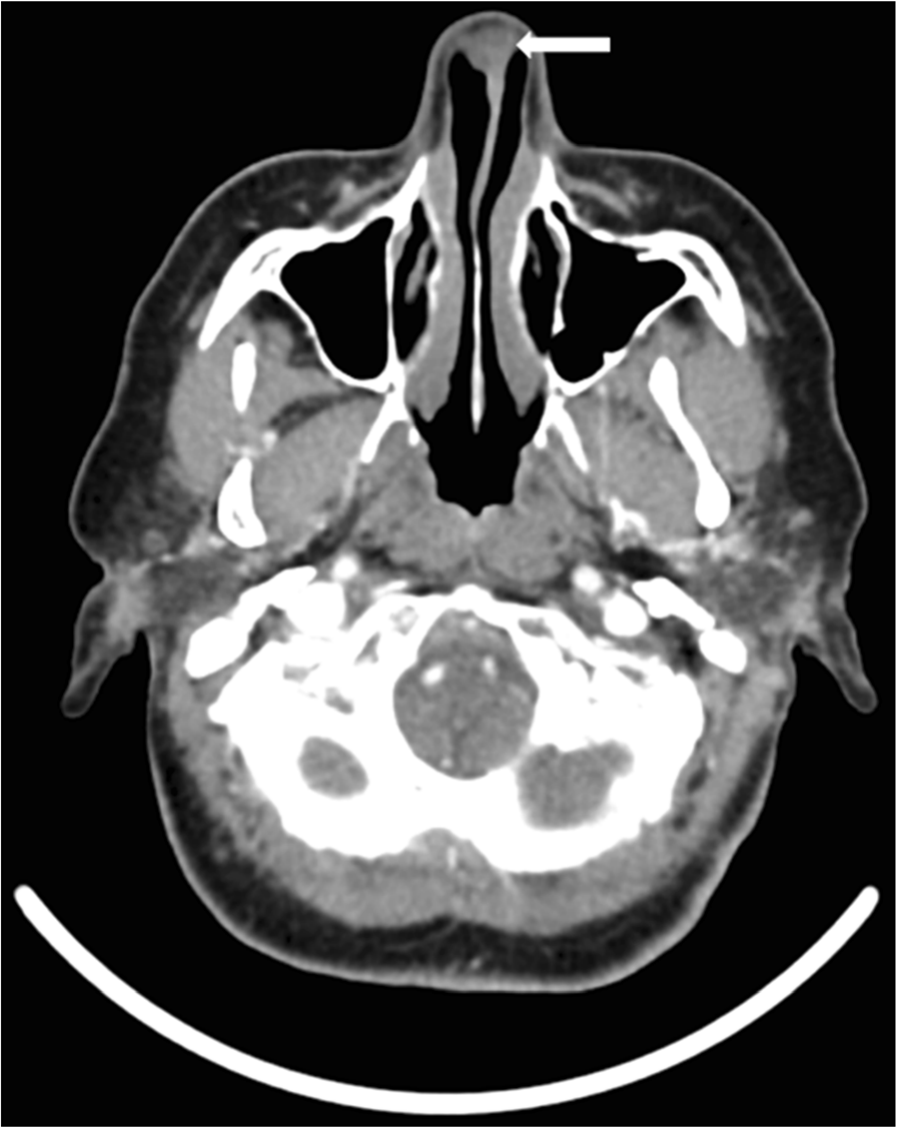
Computed tomography scan showing axial image of 2.2 × 1.5 × 1.0 cm lesion of the caudal nasal septum extending into the right and nasal cavity (arrow). No evidence of bony involvement or destructive growth
